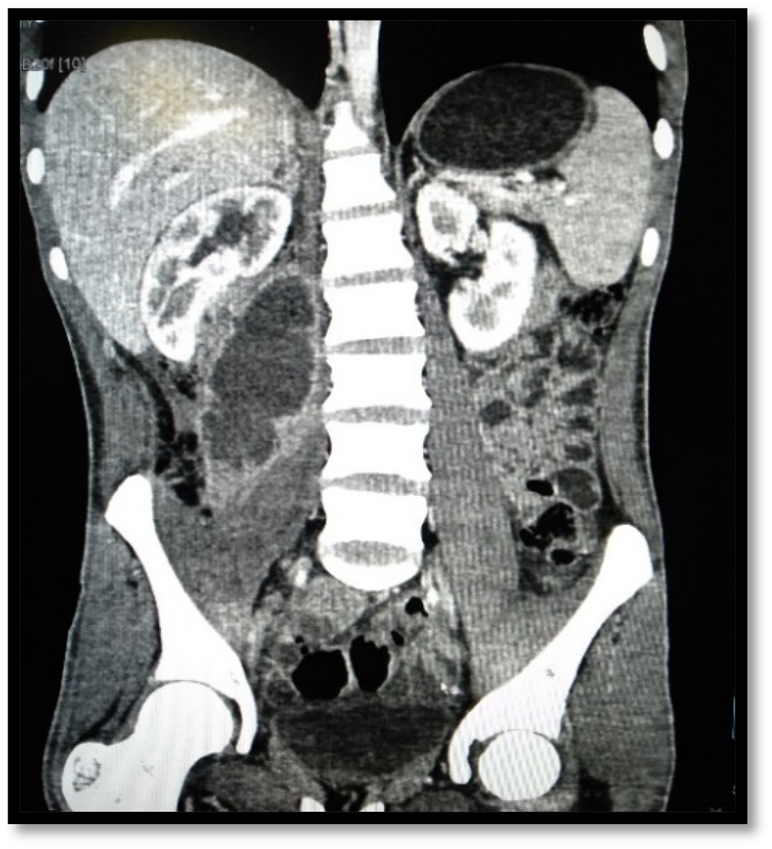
Coronal CT scan images of the abdomen and pelvis shows right sided iliopsoas abscess.
